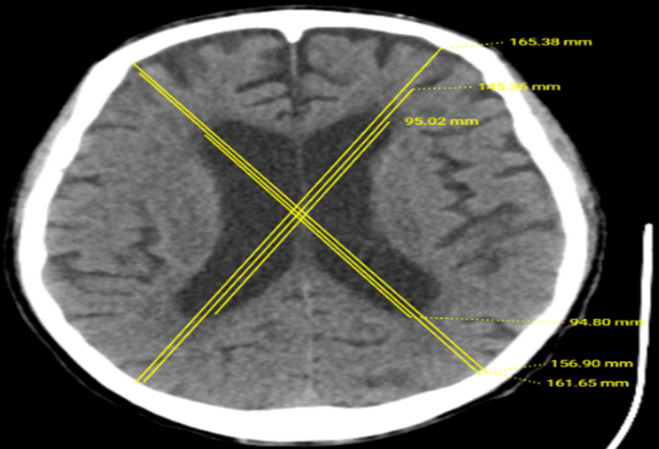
brain axial view at the level of the widest part of the lateral ventricle; CT-scan image indicating measurement as per DBF standards
